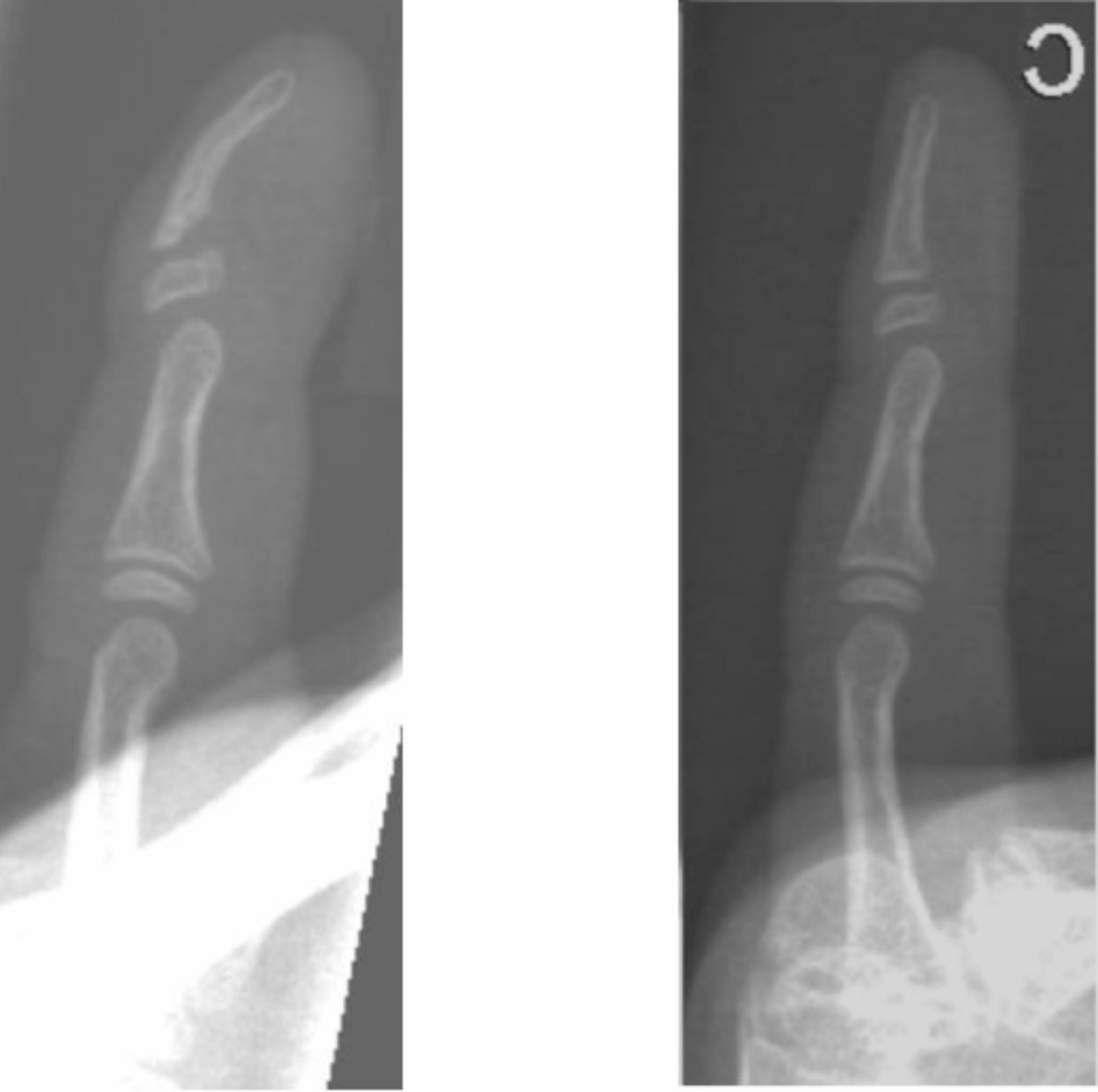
Preoperative x-ray with widened, L-shaped epiphysis with radial and palmar angulation of the distal phalanx (left); 10 months postoperative (right)
